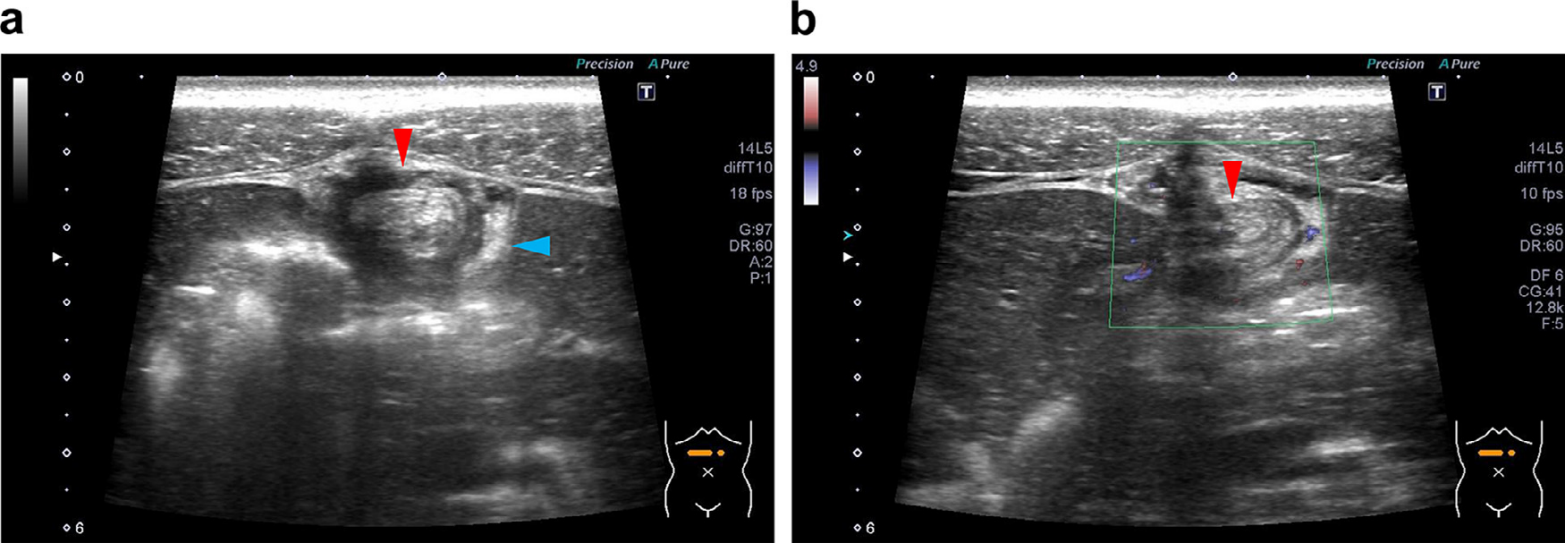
Ultrasound findings in Case 1. (A) There is a 15 × 10-mm oval-shaped hyperechoic mass adjacent to the falciform ligament with a peripherally located hypoechoic rim (red arrow head) and hyperechogenic surrounding fat (blue arrow head). (B) No internal vascularity signal is seen on Doppler evaluation.
